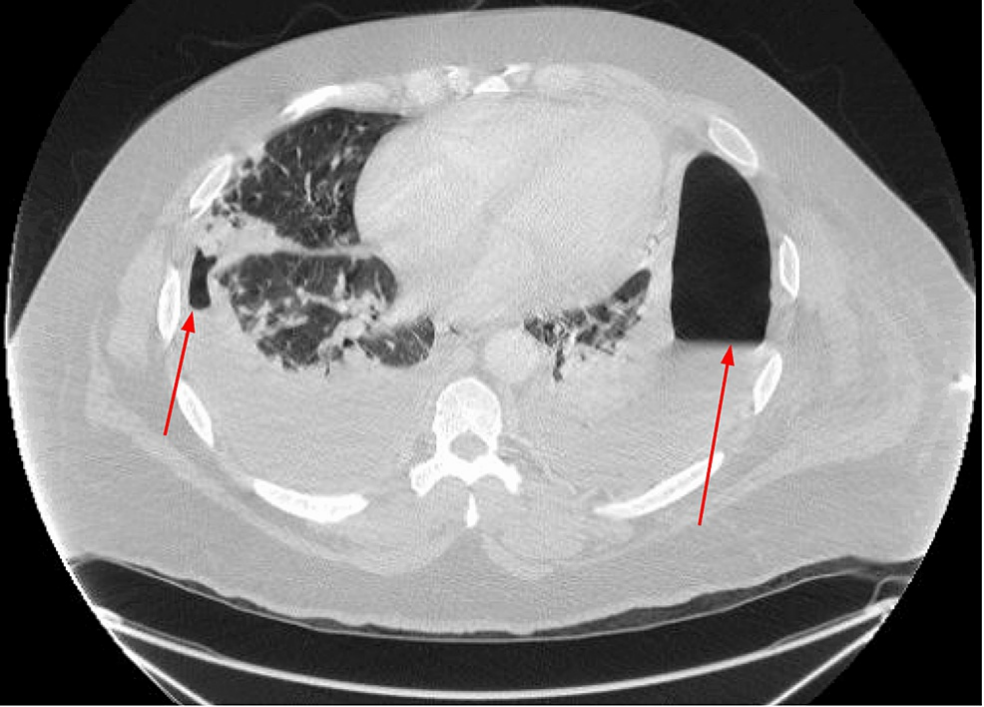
Thoracic computed tomography image demonstrating bilateral loculated pleural effusions with air in the left pleural space (red arrows).
